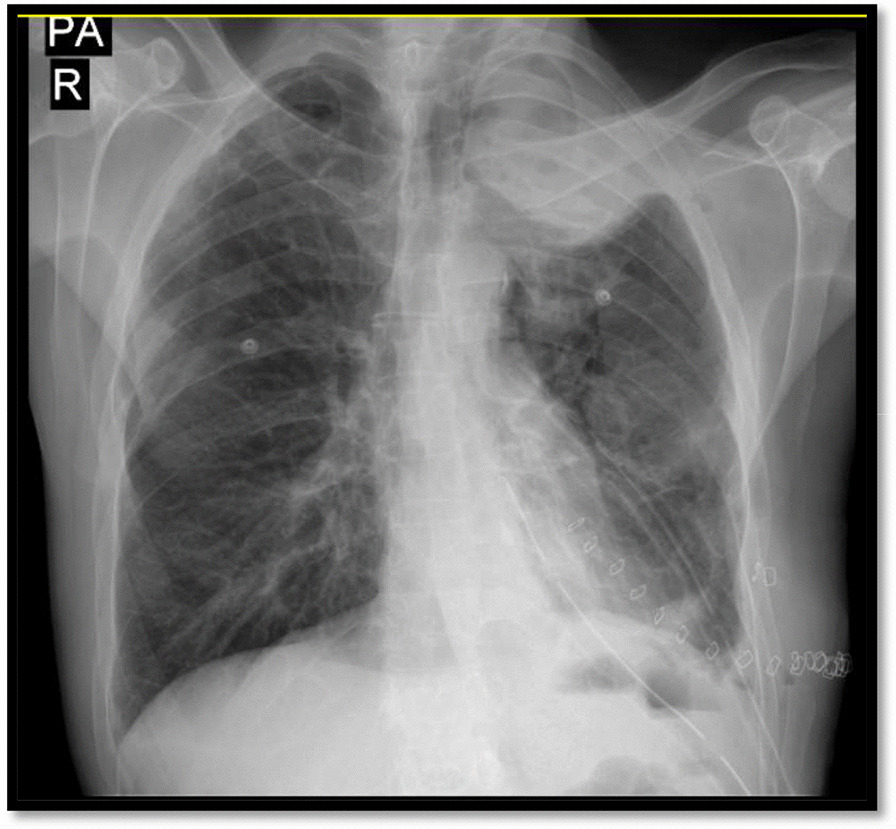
Chest radiography (2nd admission), after the operation showing two chest tubes, and resolved pneumothorax
